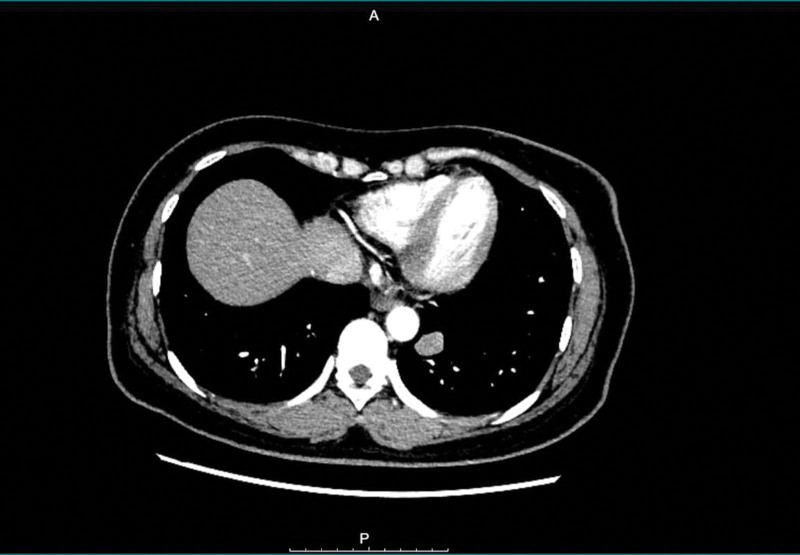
CT image indicates possible lung metastasis.
